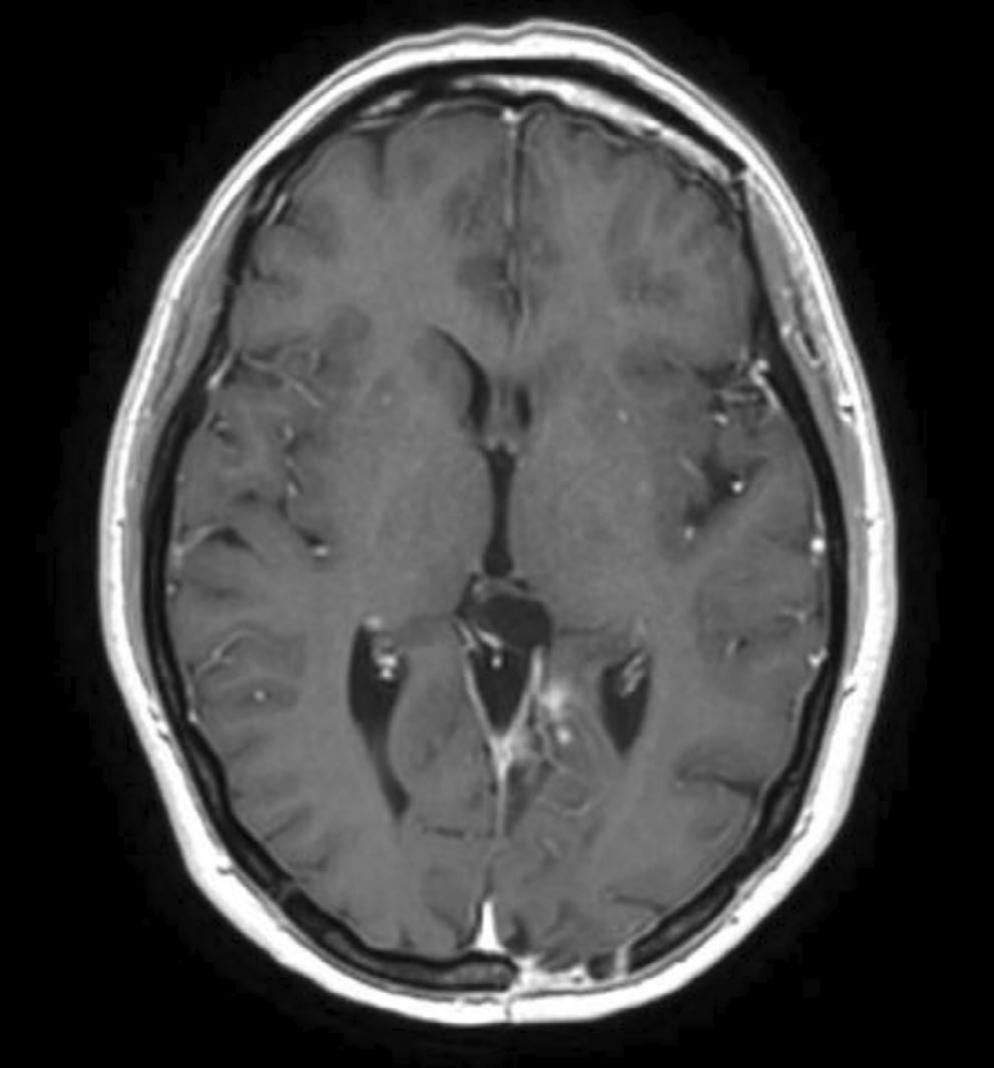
FIGURE 2 Follow up MRI
